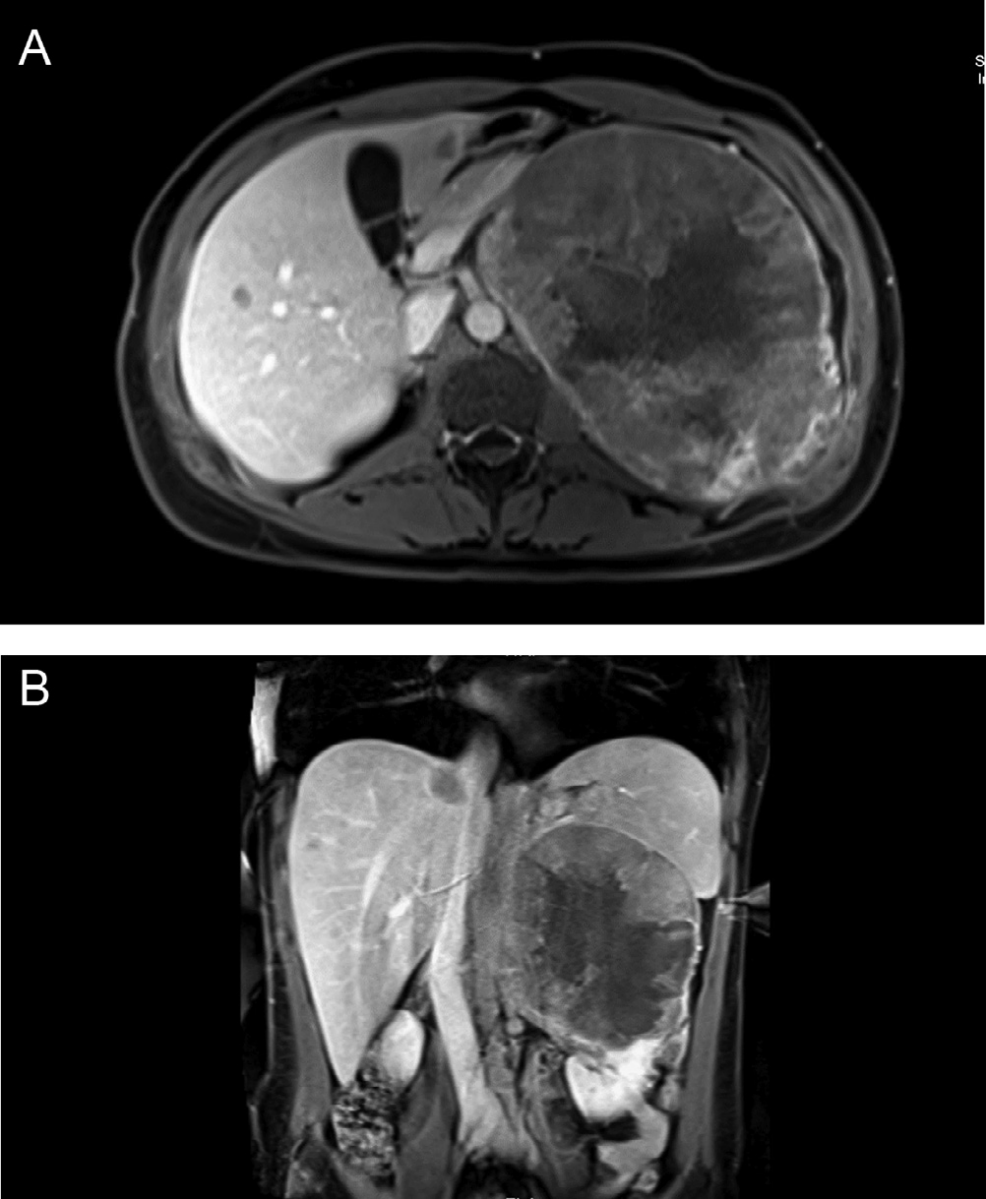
Axial (A) and coronal (B) postcontrast magnetic resonance imaging of the abdomen demonstrated a large heterogeneously enhancing mass with central necrosis arising from the left kidney.
